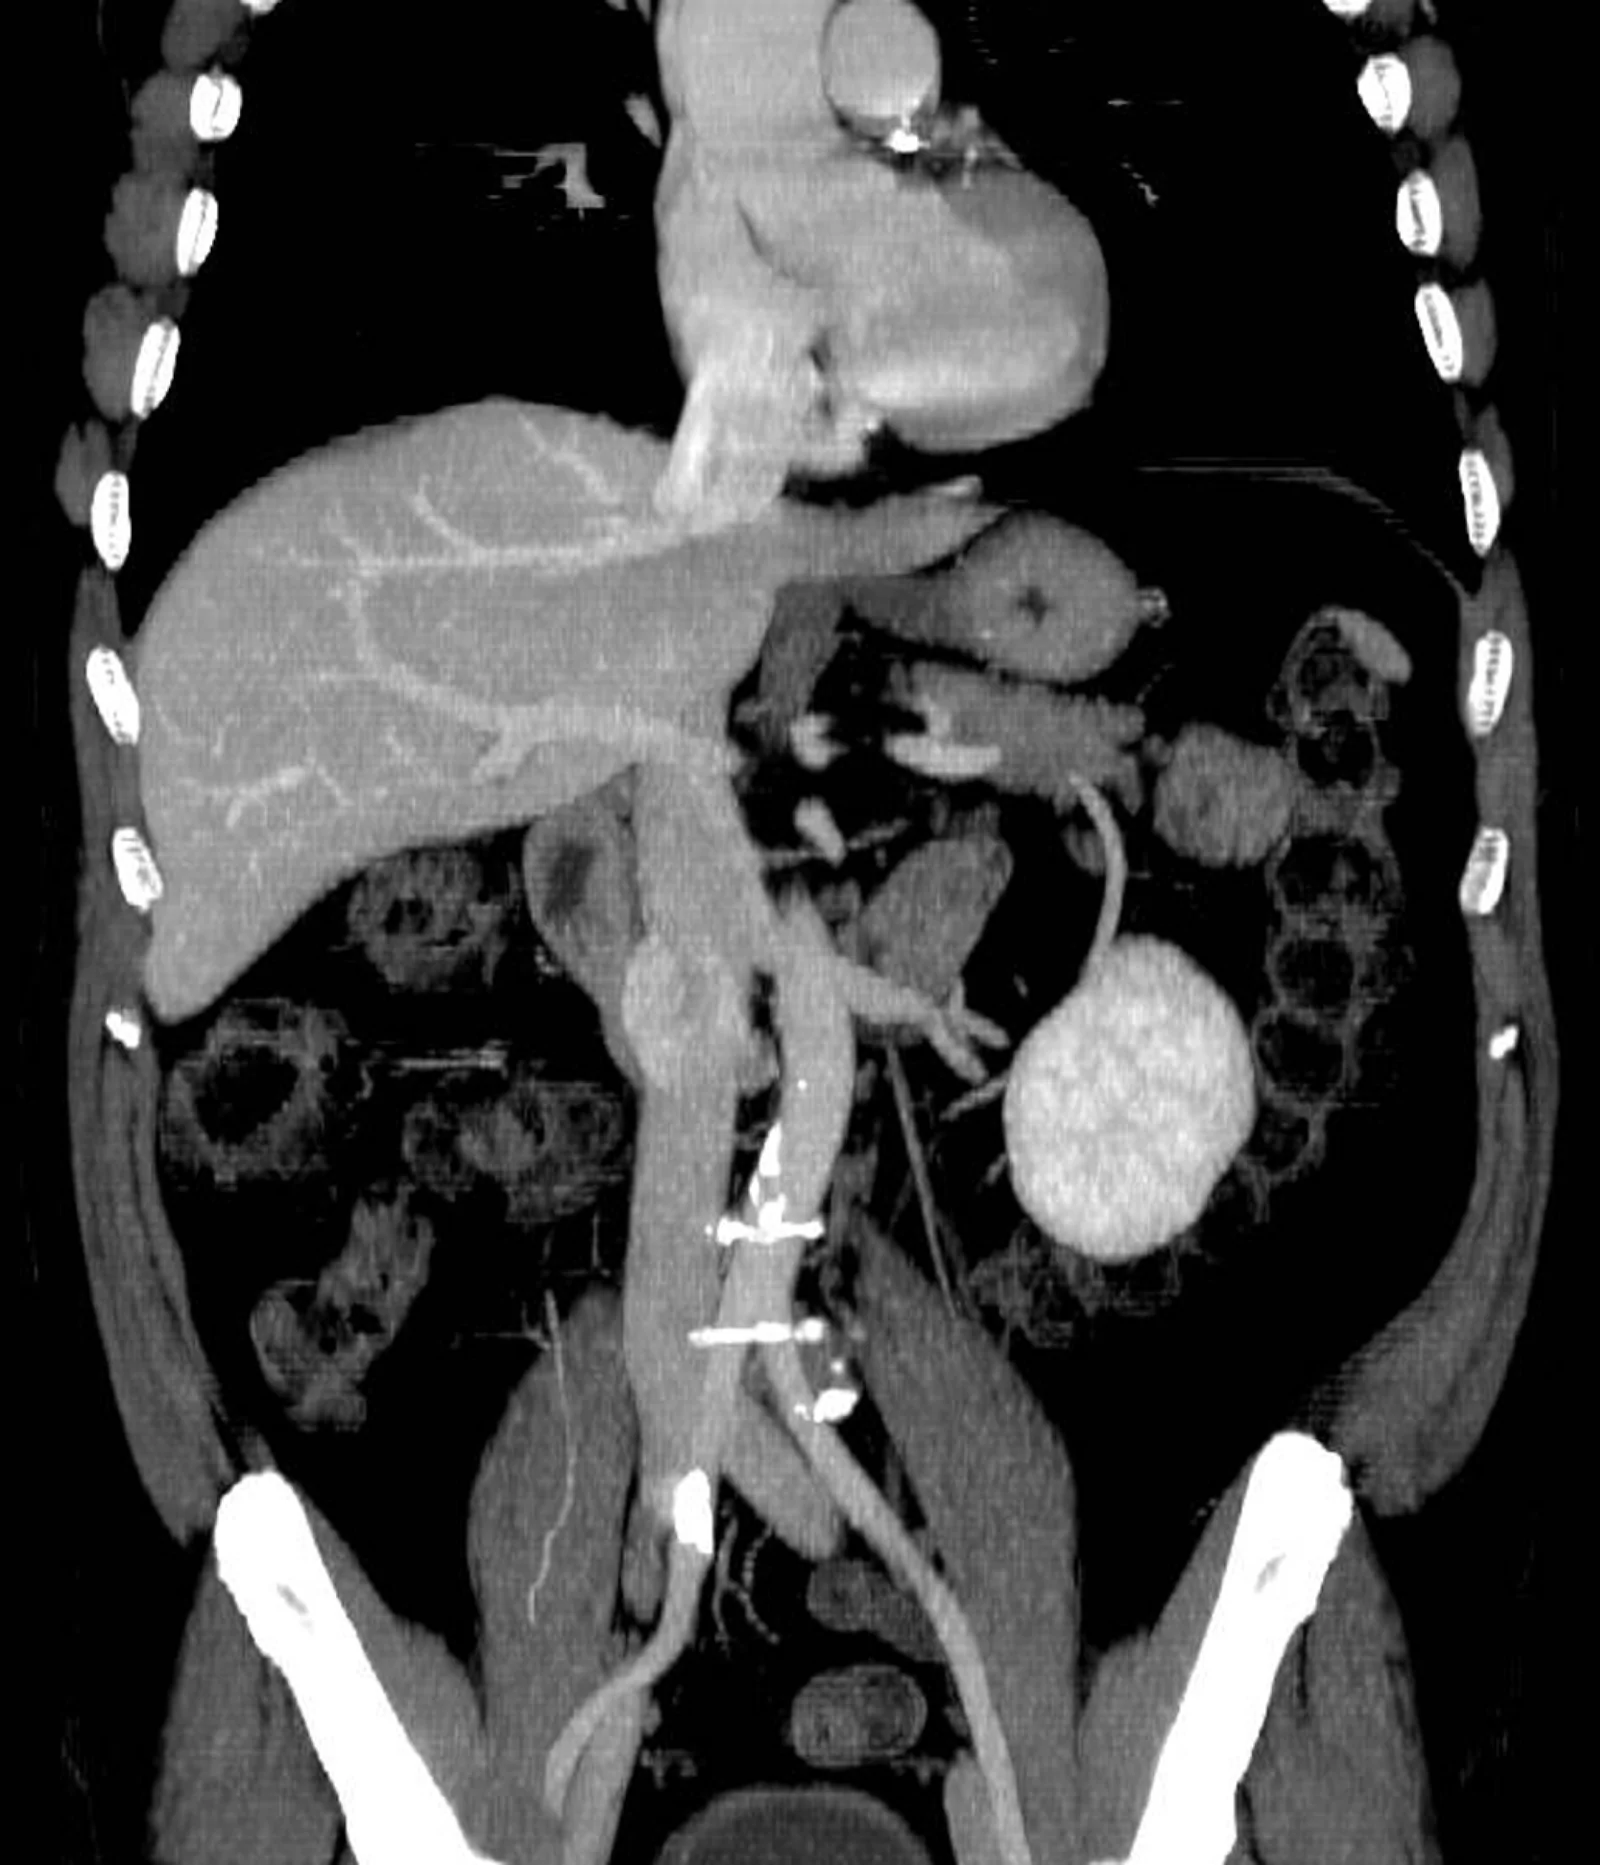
Patient’s preoperative computed tomography showing tumor recurrence involving the inferior vena cava at the level of the right renal vein stump, extending into the confluence with the left renal vein.
